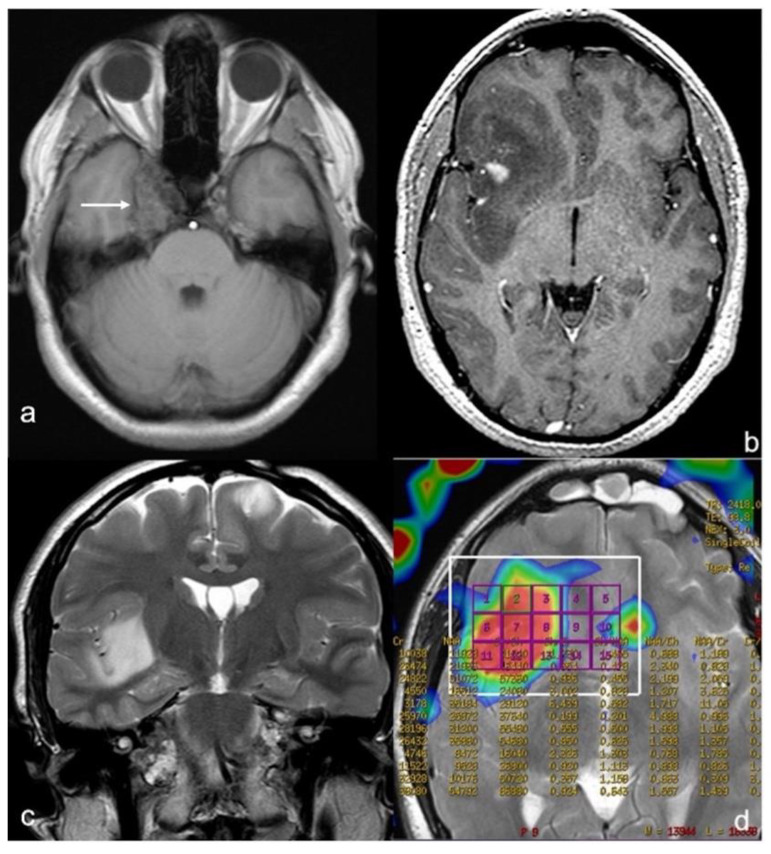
Brain MRI. (a) Axial T1 weighted sequence: bone lesion at right sphenoid wing with irregular borders to refer an enchondroma (with arrow). (b) Post-contrast axial T1-weighted sequence: multifocal bi-hemispheric lesion, with that one of maximum diameter and with contrast-enhancement at frontal-temporal-insular region of the right hemisphere. (c) Coronal T2 weighted sequence: multifocal lesion with right insular and left frontal involvement. (d) Perfusion axial sequence: increased cerebral blood flow at the lesion.
